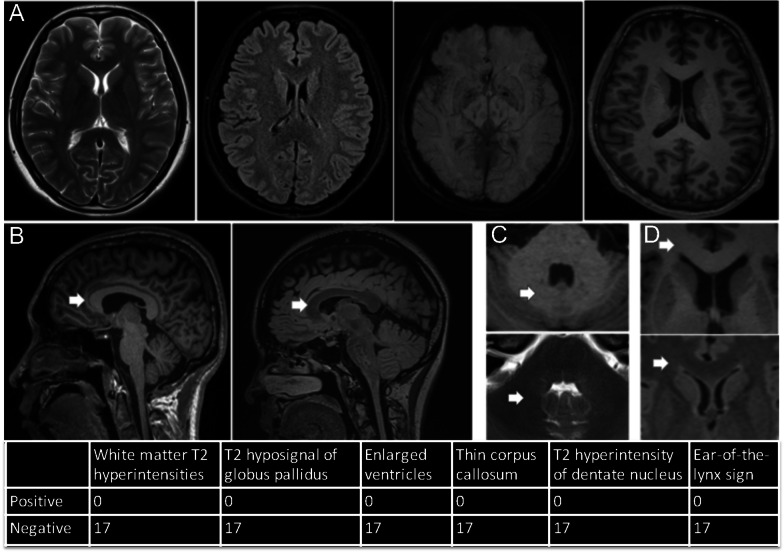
Conventional brain MRI evaluation. A Abnormal signals were assessed on T2, FLAIR, SWI and T1 routine sequence (above panel, from left to right). B–D Neuroradiological signs, previously reported in other subtypes of hereditary spastic paraplegias, were also assessed; B thin corpus callosum (left T1, right T2 FLAIR, green edge arrow); C T2 hyperintensity of the dentate nucleus (above T1, below T2; black edge arrow); D “Ears- of -the- Lynx” MRI sign (above T1, below T2 FLAIR, red edge arrow)
